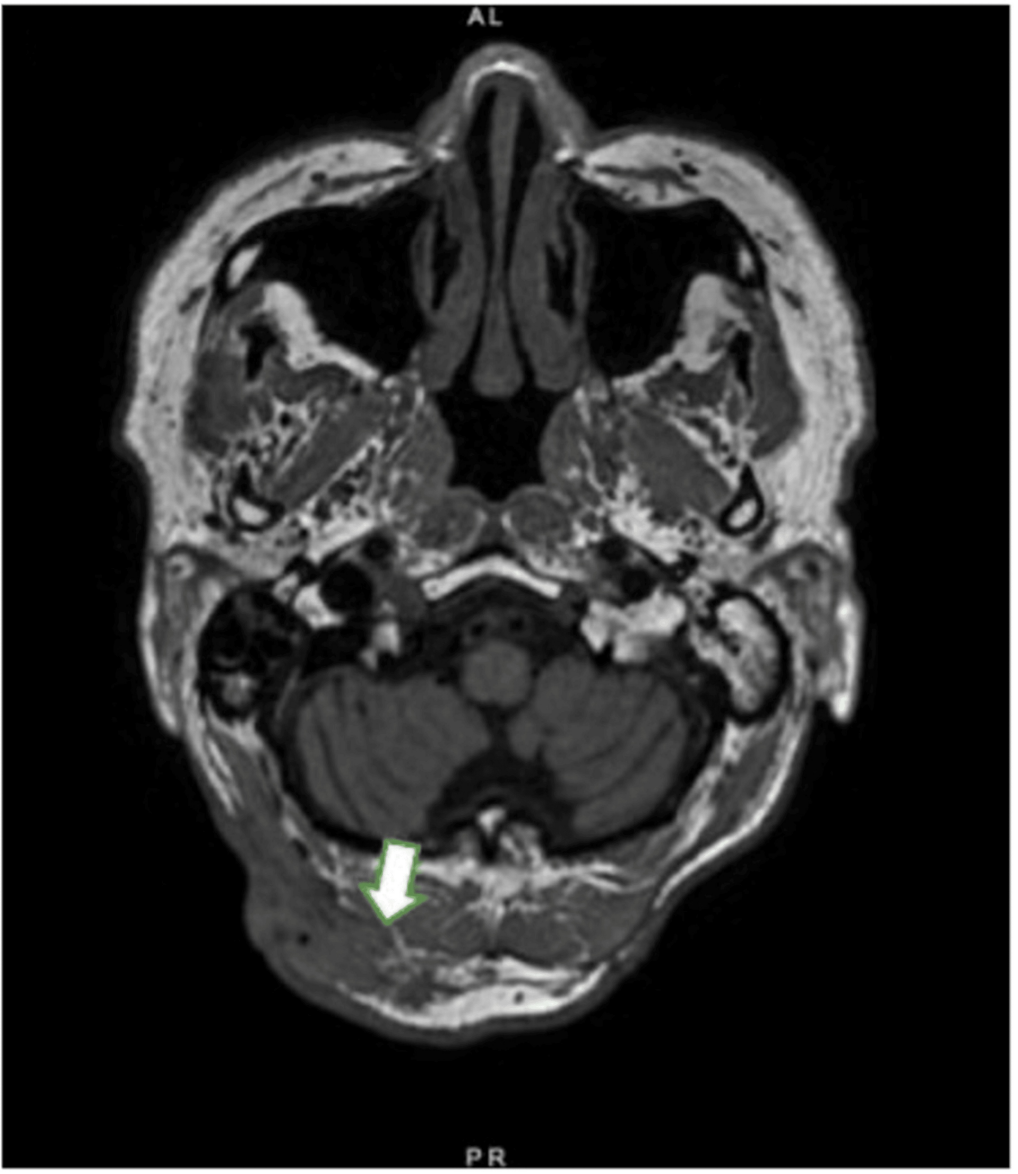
Suboccipital soft tissue mass The arrow in T1 magnetic resonance imaging (MRI) points to the 2.1 x 1.6 x 4.6 cm irregular enhancing right suboccipital soft tissue mass infiltrating the fascia and abutting the right splenius capitis and rectus capitis.
